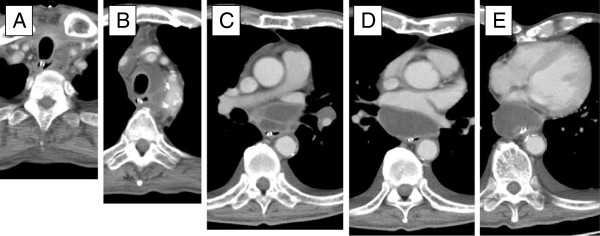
Chest CT scan on admission. From head to abdomen (A to E), low-density masses in the mediastinal region are recognizable in all scans.
